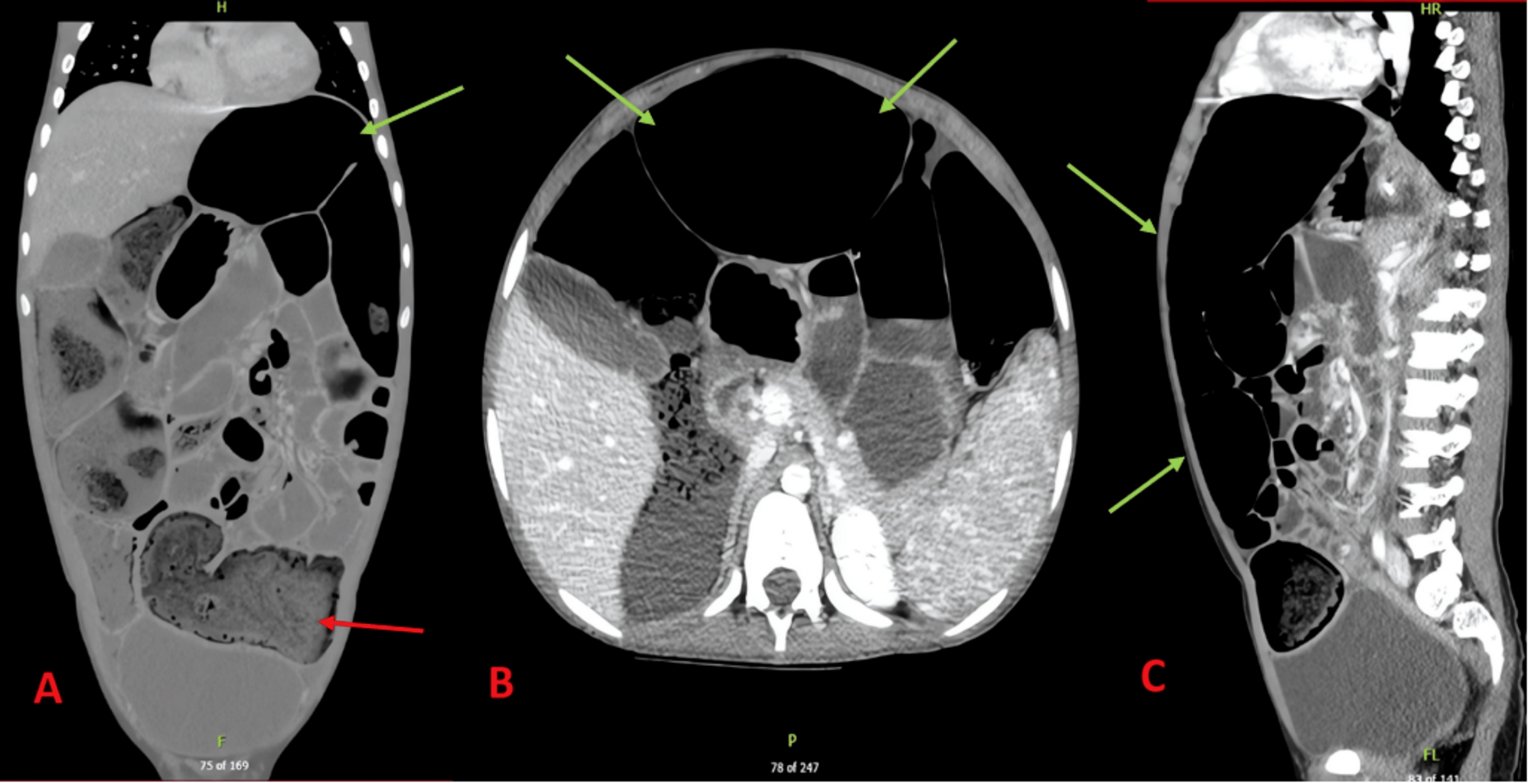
Contrast-Enhanced CT Findings (A) Coronal contrast-enhanced CT showing extensive air- and fluid-filled dilated bowel loops (green arrows). (B) Axial contrast-enhanced CT demonstrating pneumatosis (red arrows) without signs of transmural ischemia. (C) Sagittal contrast-enhanced CT displaying marked colonic distention (green arrows) and bowel wall edema.
